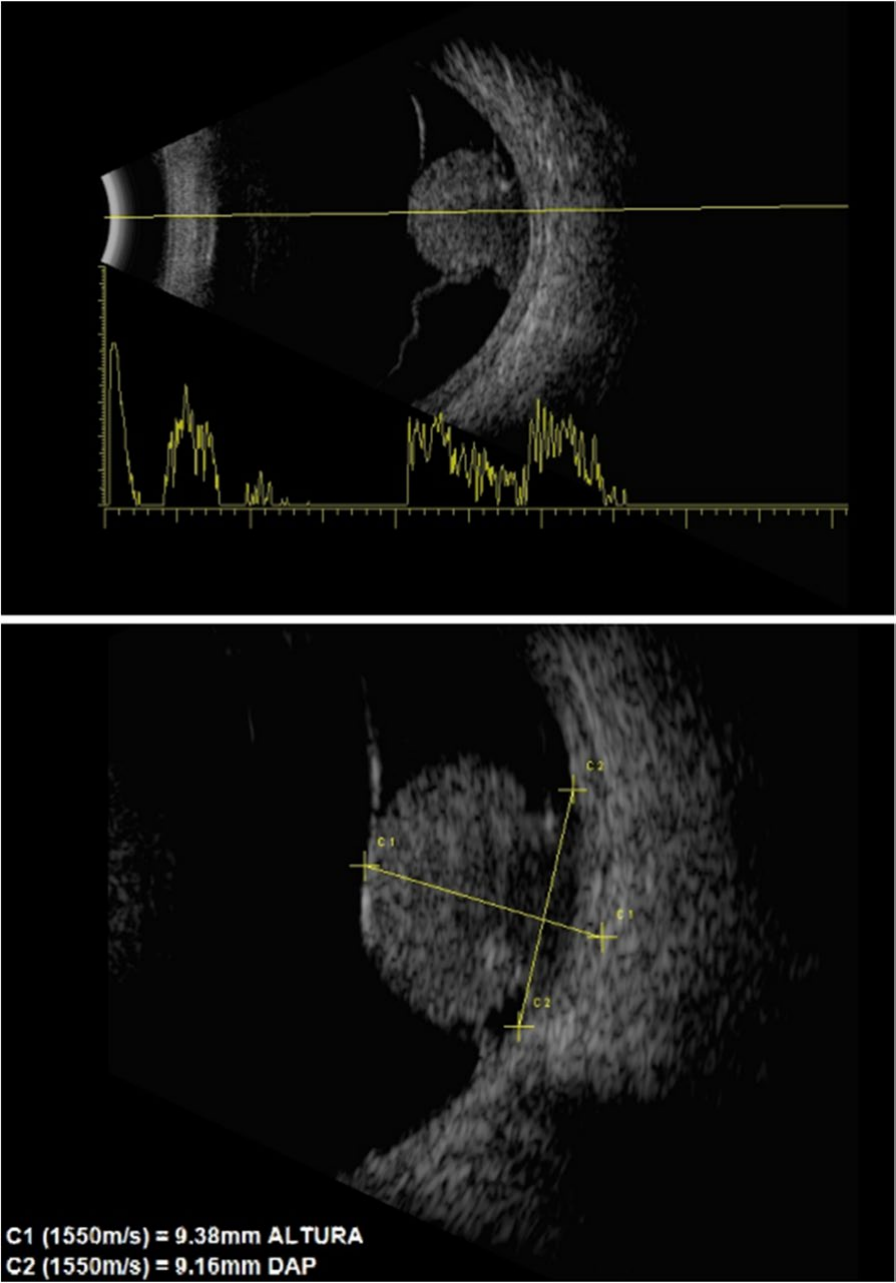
Ocular ultrasound from a 16 years old female patient with a mushroom shaped choroidal melanoma. Superior image shows a transversal B-scan on posterior pole – the lesion has medium to low reflectivity decreasing towards the tumor base, indicating kappa angle sign. Inferior image shows a longitudinal B-scan – the lesion presents acoustic hollowness at its base, tumor dimensions were 9.38 mm of height and 9.16 mm of circumferential basal diameter
